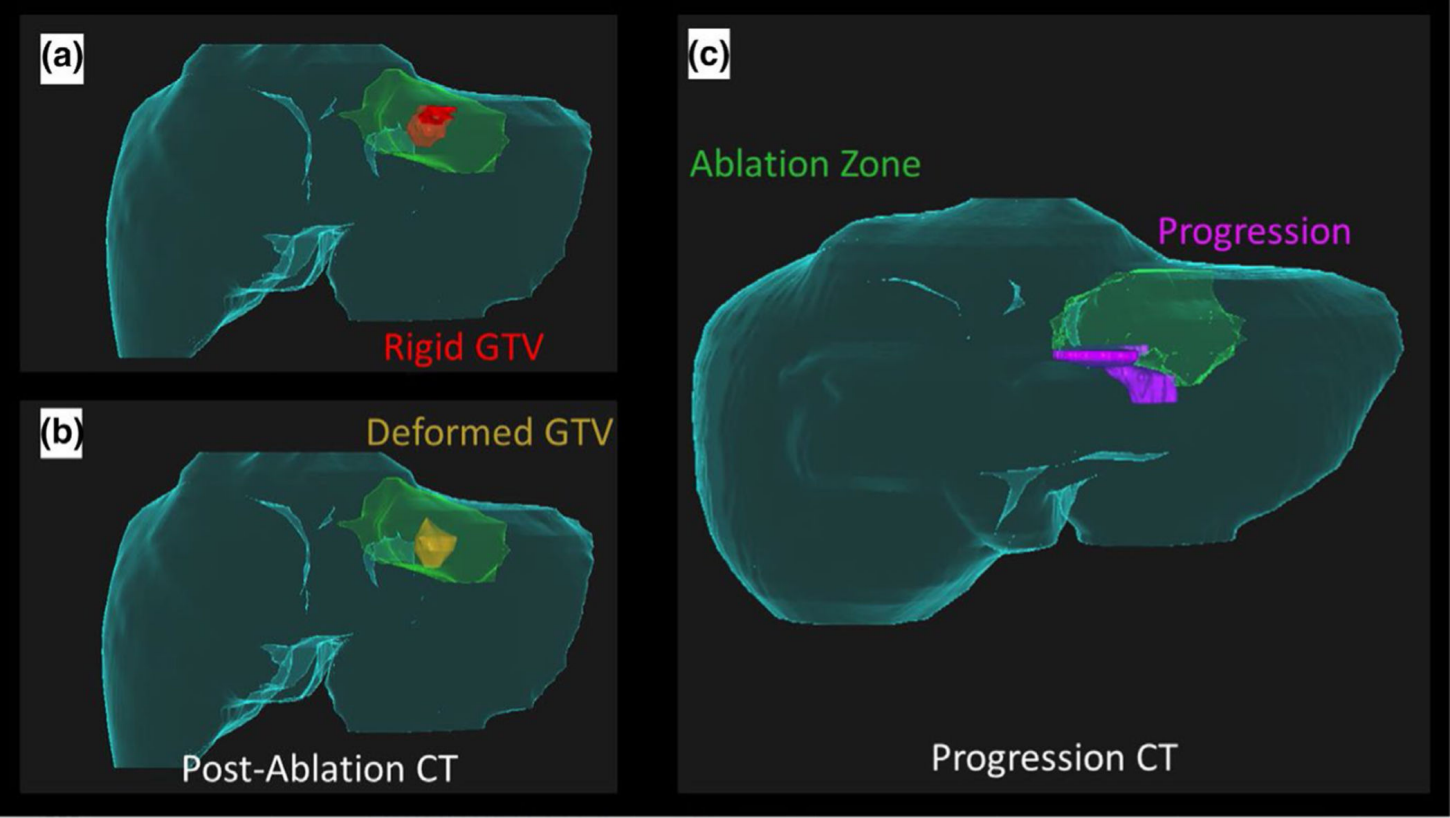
Failings of rigid registration. (a) Rigid registration of the gross tumor volume (GTV, red) onto the post-ablation CT maps part of the tumor outside of the ablation zone (green), and gives a false sense of confidence in the delivered margin caudally. (b) Deformable registration of the GTV (gold) onto the post-ablation CT indicates potentially insufficient ablation margin in caudal aspect. (c) Development of disease progression (pink) in the caudal aspect of the recovered ablation zone (green) corresponds with insufficient margin in (b)
